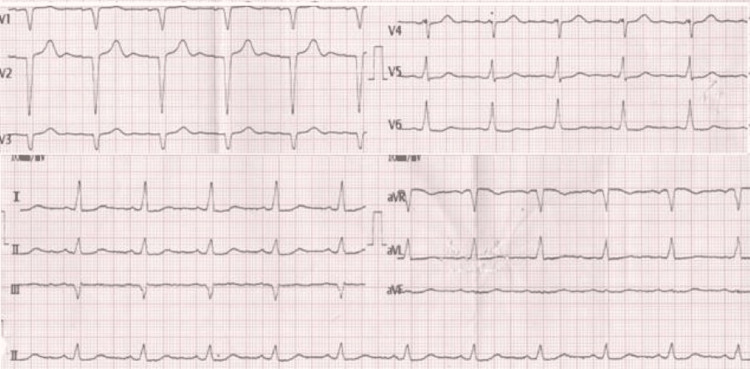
Echocardiogram on admission showing normal sinus rhythm with no ischemic lesions.
